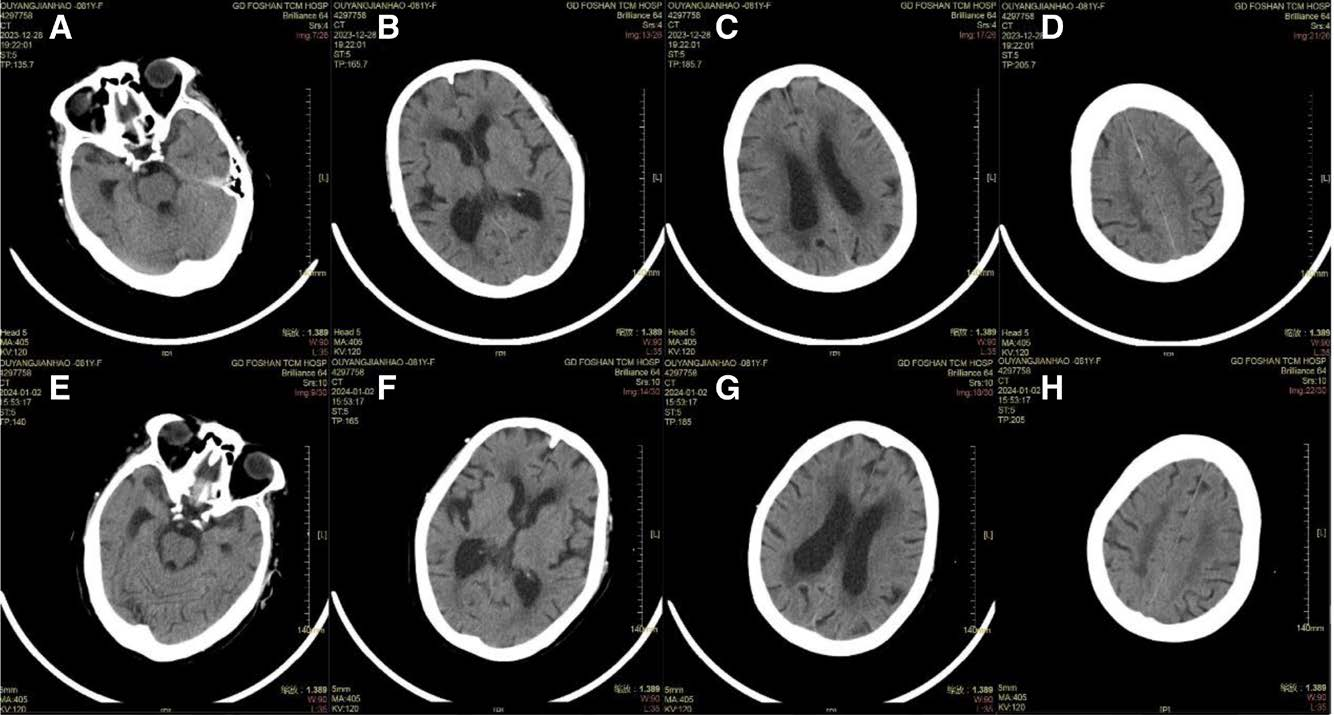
Patient’s CT. (A–D) shows cranial CT on December 28, 2023, suggesting cerebral atrophy and cerebral white matter sparing. (E and F) shows cranial CT on January 2, 2024, suggesting focal cerebral infarction in the right thalamus and right occipital lobe. CT = computed tomography.
